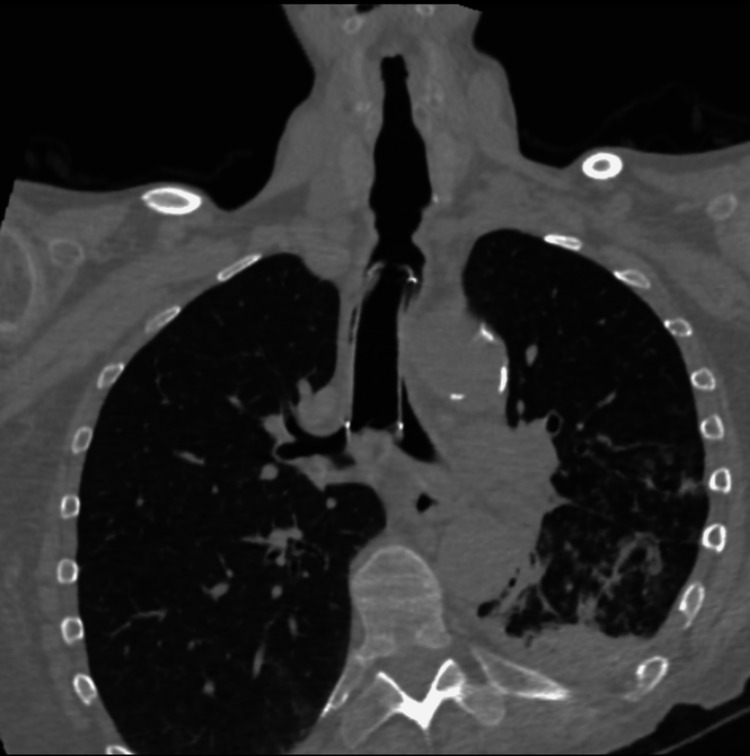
Coronal CT view of the anatomical relationships of the stent.
